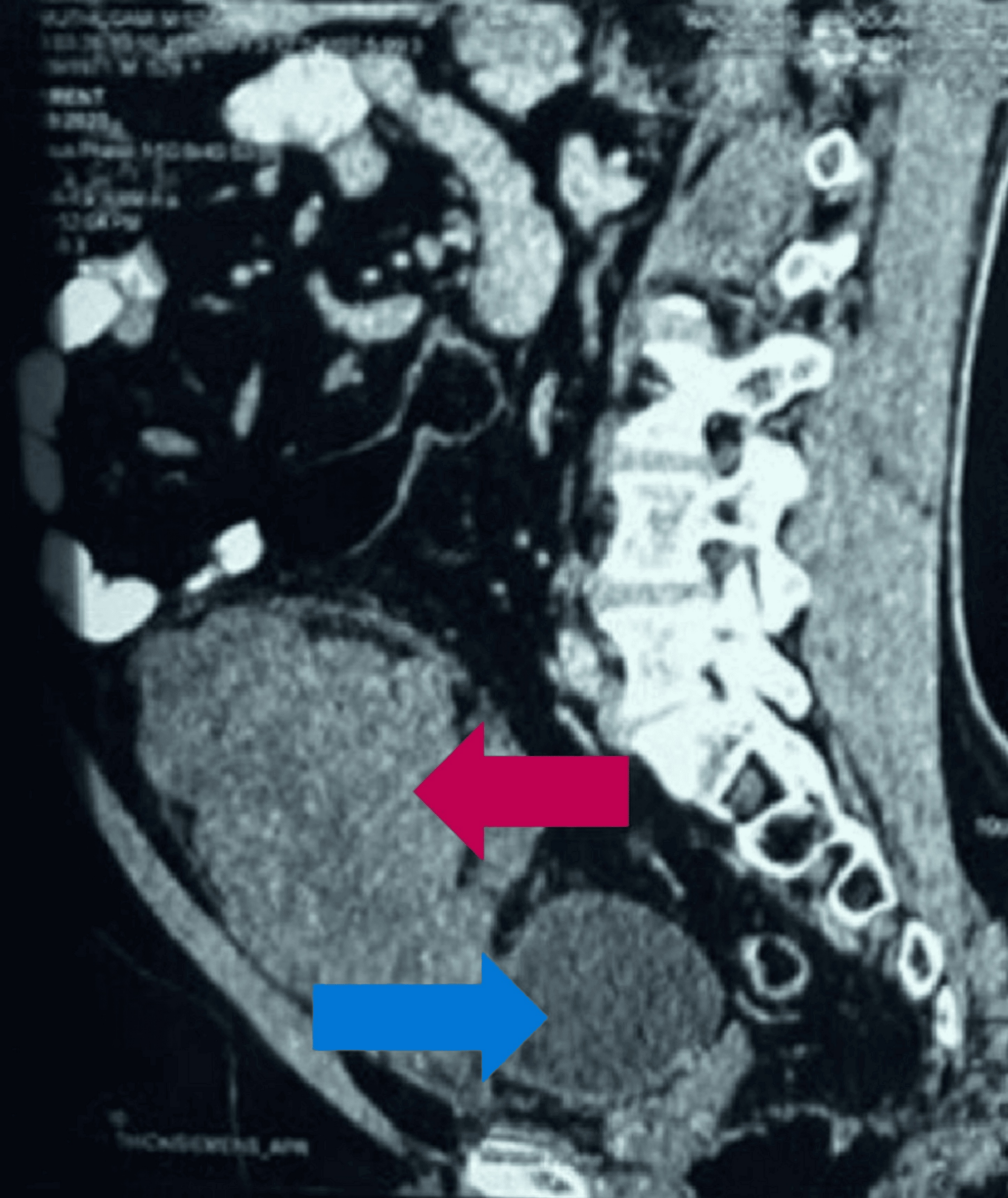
Sagittal section of the CT abdominal scan, showing the tumor (red arrow) arising from posterior to the anterior abdominal wall without any infiltration to the urinary bladder (blue arrow) CT: computed tomography
